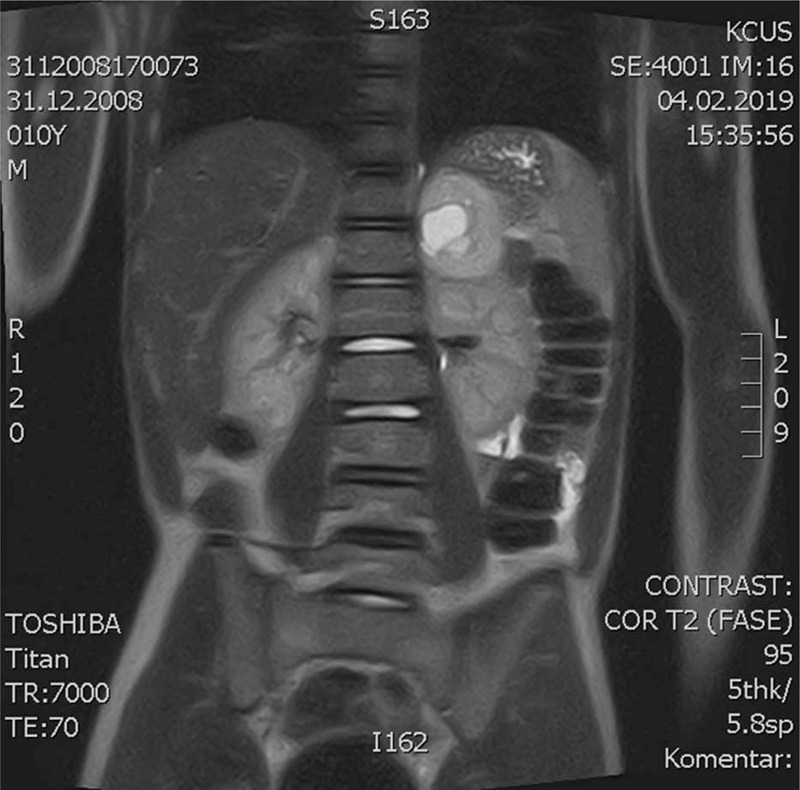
An abdominal magnetic resonance imaging revealed the left adrenal mass measuring 47.5 × 46.4 × 42 mm.
